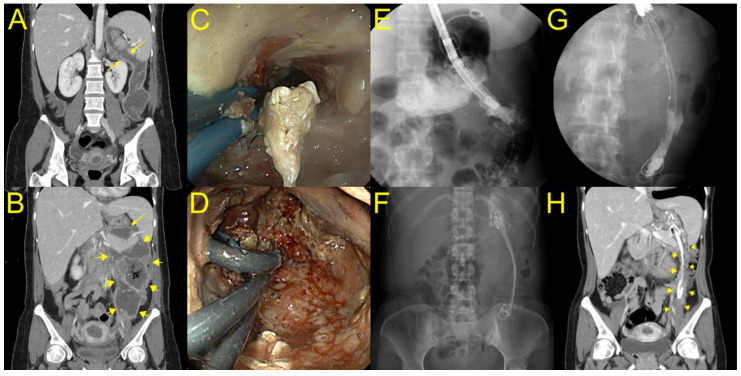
A case of elongated WON extended to the left paracolic gutter. (A,B) Computed tomography showing that an elongated WON (yellow arrow) extended to the left paracolic gutter was concatenated with the stomach. (C–E) A gastroscope was inserted into the proximal portion of the cavity through a lumen-apposing metal stent (LAMS), and we found a long narrowing WON endoscopically and radiologically. (F) After DEN, three plastic stents with double pigtail configuration remained in place through LAMS to the deep portion of WON until the next session of DEN. (G) After nine DEN procedures, long plastic stents remained in the deep portion of WON with partial improvement radiologically. (H) After 11 DEN sessions, where the long plastic stents remained in the deep portion of WON, the patient recovered with complete WON resolution (yellow arrow).
